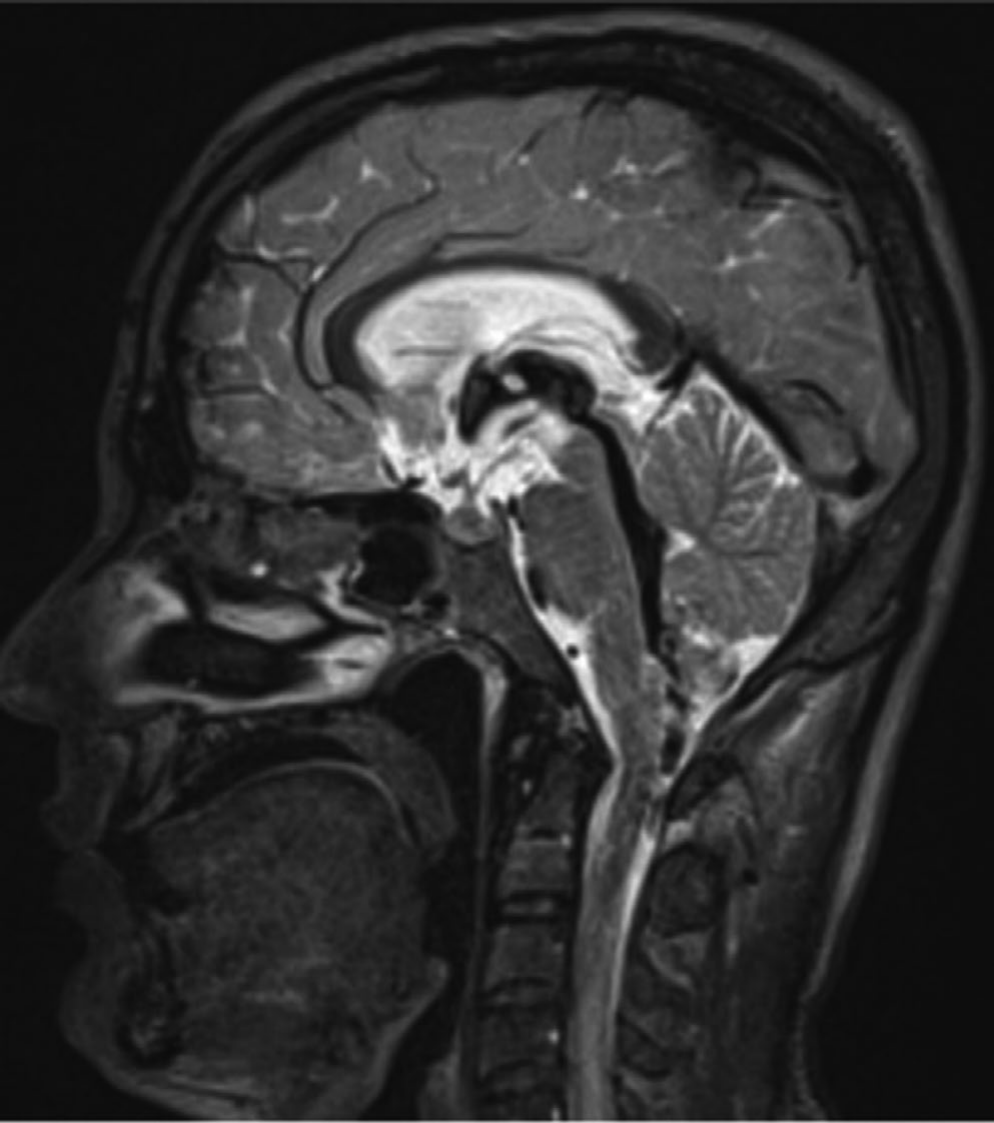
Sagittal T2‐weighted MR image demonstrates altered signal intensity of the spinal cord, dilatation of the cerebral ventricles
